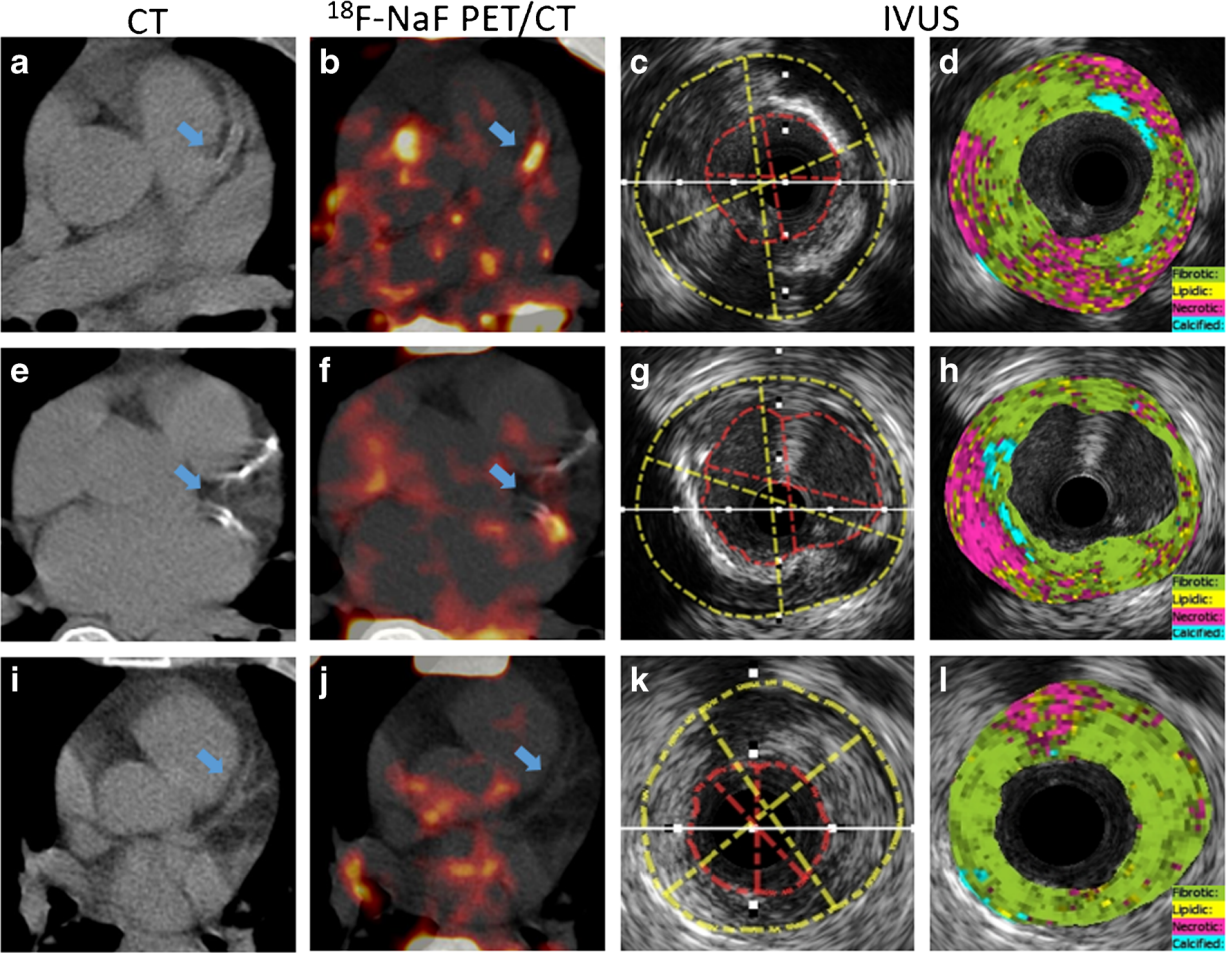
Representative images of PET/CT and IVUS from three patients with unstable angina. Increased ^18^F-NaF focal uptake was seen at atheromatous lesions with spotty calcification (a–d) in segments of the LAD, and thin-cap fibrocalcified lesions with a large necrotic core (e–h) in segments of the LCX. But, there was no uptake in fibrotic lesions (i–l) in segments of the LAD
